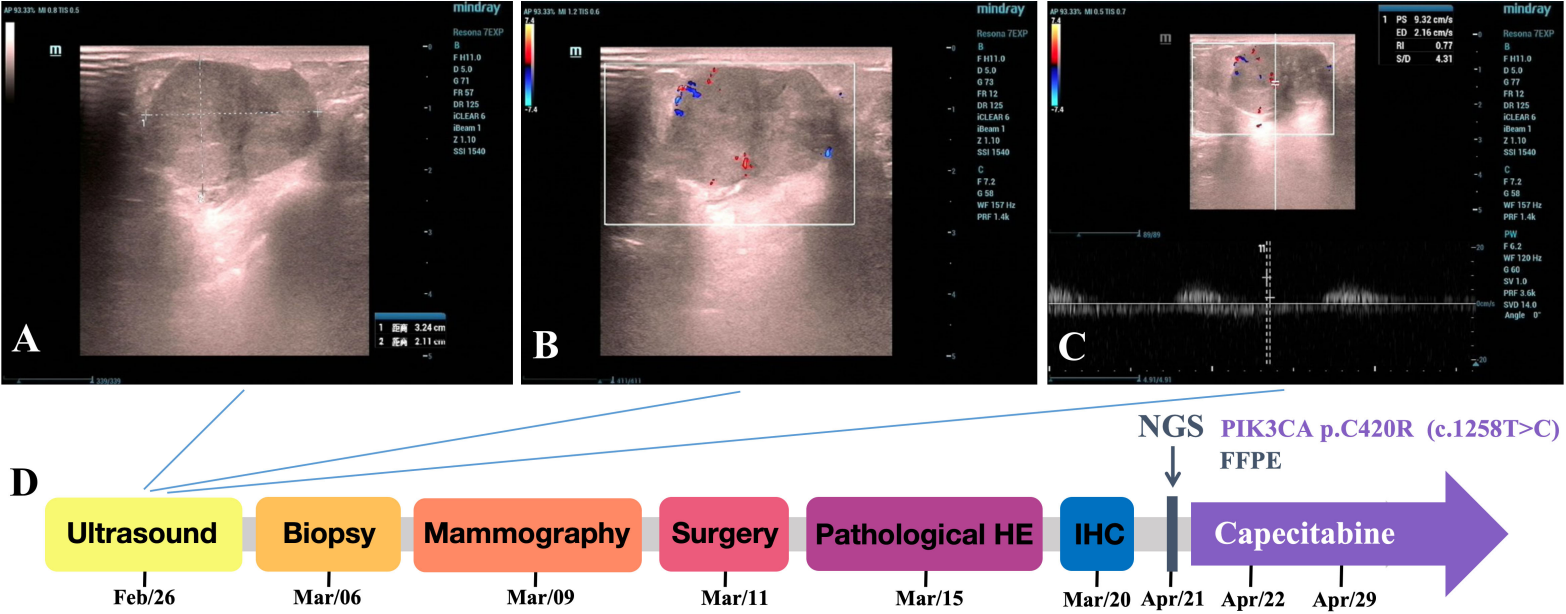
The two-dimensional ultrasound image of the 3.2×2.1 cm hypoechoic mass has relatively clear boundaries, regular morphology, slightly irregular margins, and heterogeneous internal echogenicity. (B) The color Doppler ultrasound image showing detectable blood flow signals within the mass. (C) The spectral Doppler image demonstrating a resistance index (RI) of 0.77. (D) Disease time line showed the various treatment received by the patient.
